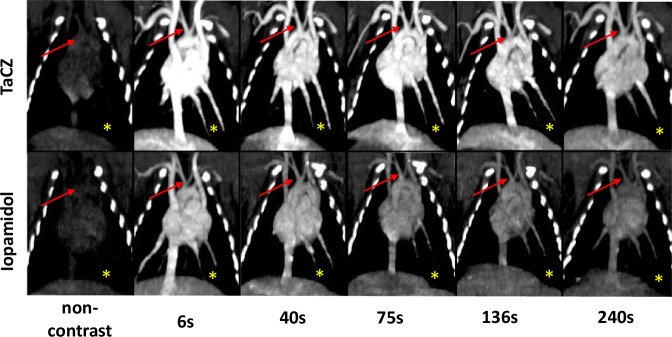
TaCZ improves thoracic vascular contrast with superior conspicuity at CTA window levels. Vascular contrast enhancement of the heart and large thoracic vasculature. Representative coronal maximum intensity projection at 10 mm before and 6 s, 40 s, 75 s, 136 s, and 240 s after intravenous administration of TaCZ (top row) or Iopamidol (bottom row) at CTA window levels (W/L = 300/170 HU). The heart and larger thoracic vessels are displayed; the brachiocephalic trunk (red arrow) and left inferior pulmonary artery and vein (yellow asterisk) are highlighted. Overall, TaCZ provides superior contrast enhancement at all scan delays with sustained vascular enhancement at 240 s after contrast administration. Parenchymal liver enhancement is also increased in the TaCZ cohort. CTA, Computed tomography angiography; L, Level; TaCZ, Carboxybetaine zwitterionic tantalum oxide; W, Width
